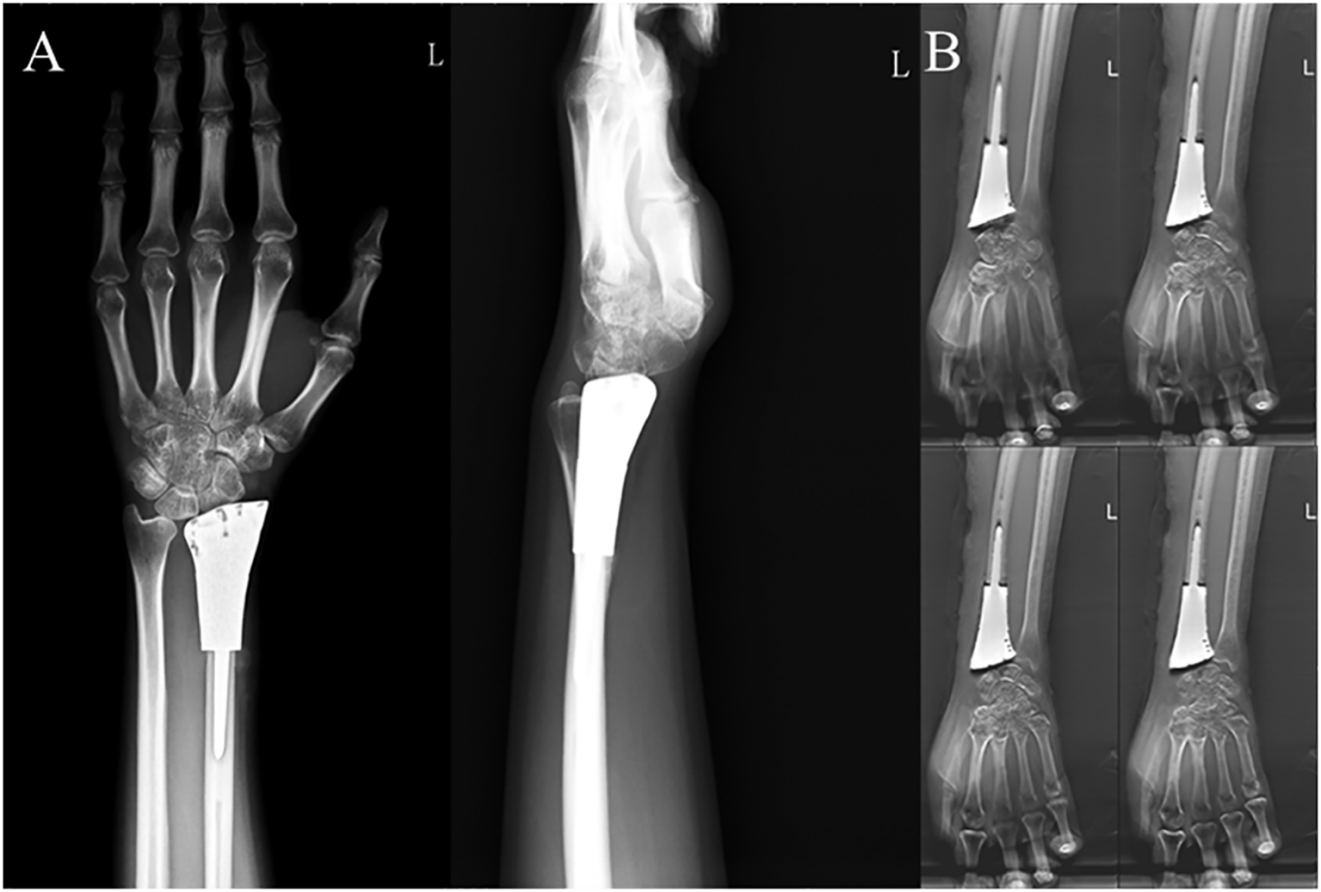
Postoperative examination results of a typical patient. (A) Anteroposterior and lateral X-rays of the wrist joint and (B) T-SMART six months after 3D-printed customized biological prosthesis replacement surgery.
